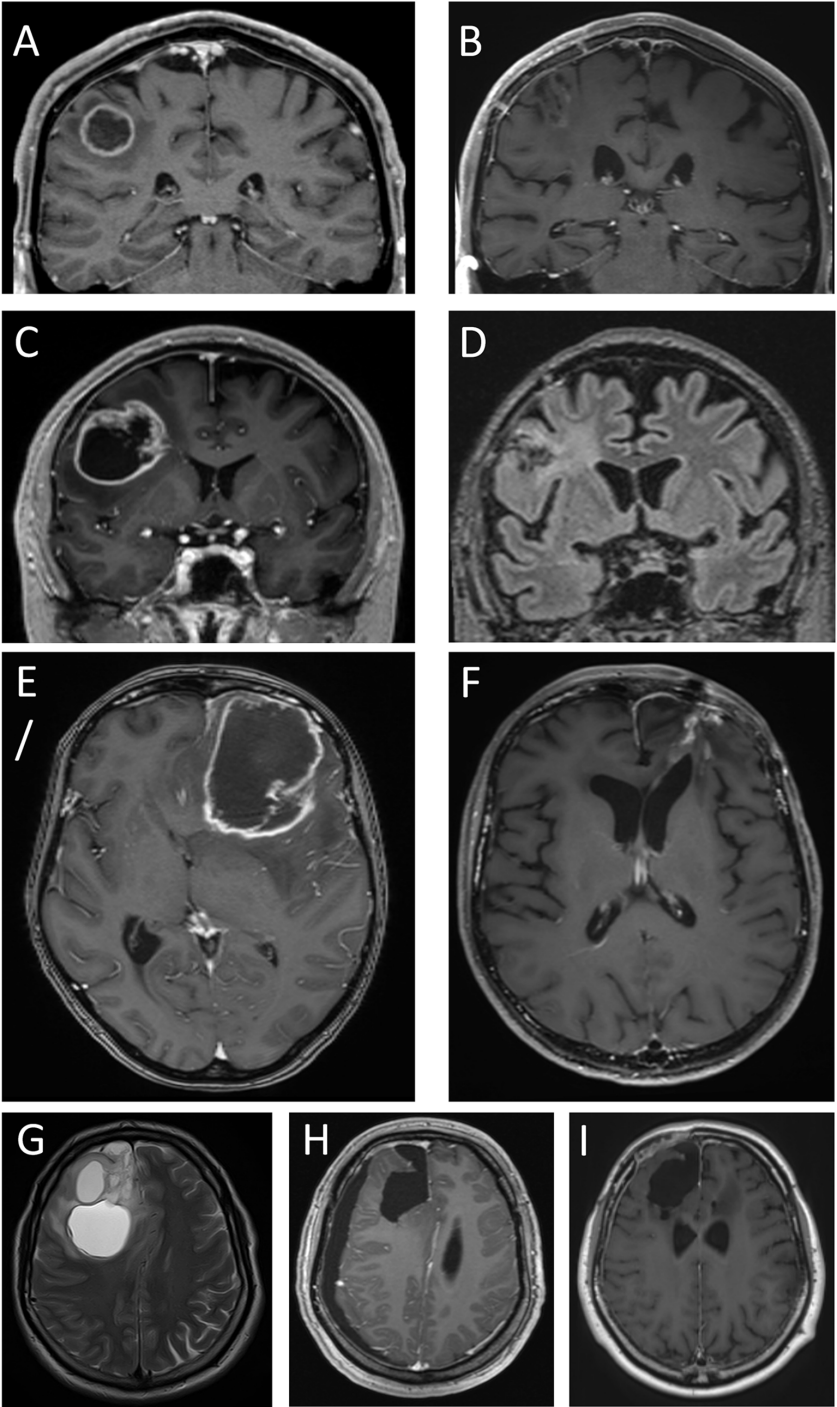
Four cystic glioblastomas before and after tumor surgery. T1 weighted MPRAGE MRIs after intravenous injection of gadolinium-based contrast medium (which gives the ring-formed bright signal in most images), except in D and G, which are T2-weighted and T2-weighted FLAIR, respectively. Images (A, C, E, G) show pre-surgical MRIs. Note the modest displacement of surrounding brain tissue (compare with contralateral side). Images (B, D, F, H, I) show MRIs after 240, 295, 330, 39 days, and 45 months, respectively. Tumors in images (A, C) were assessed as having minimal mass effect, tumors in images (E, G) had pronounced mass effect. Note the absence of brain tissue where the tumor and cyst resided; this comprises white matter and adjacent neocortex.
